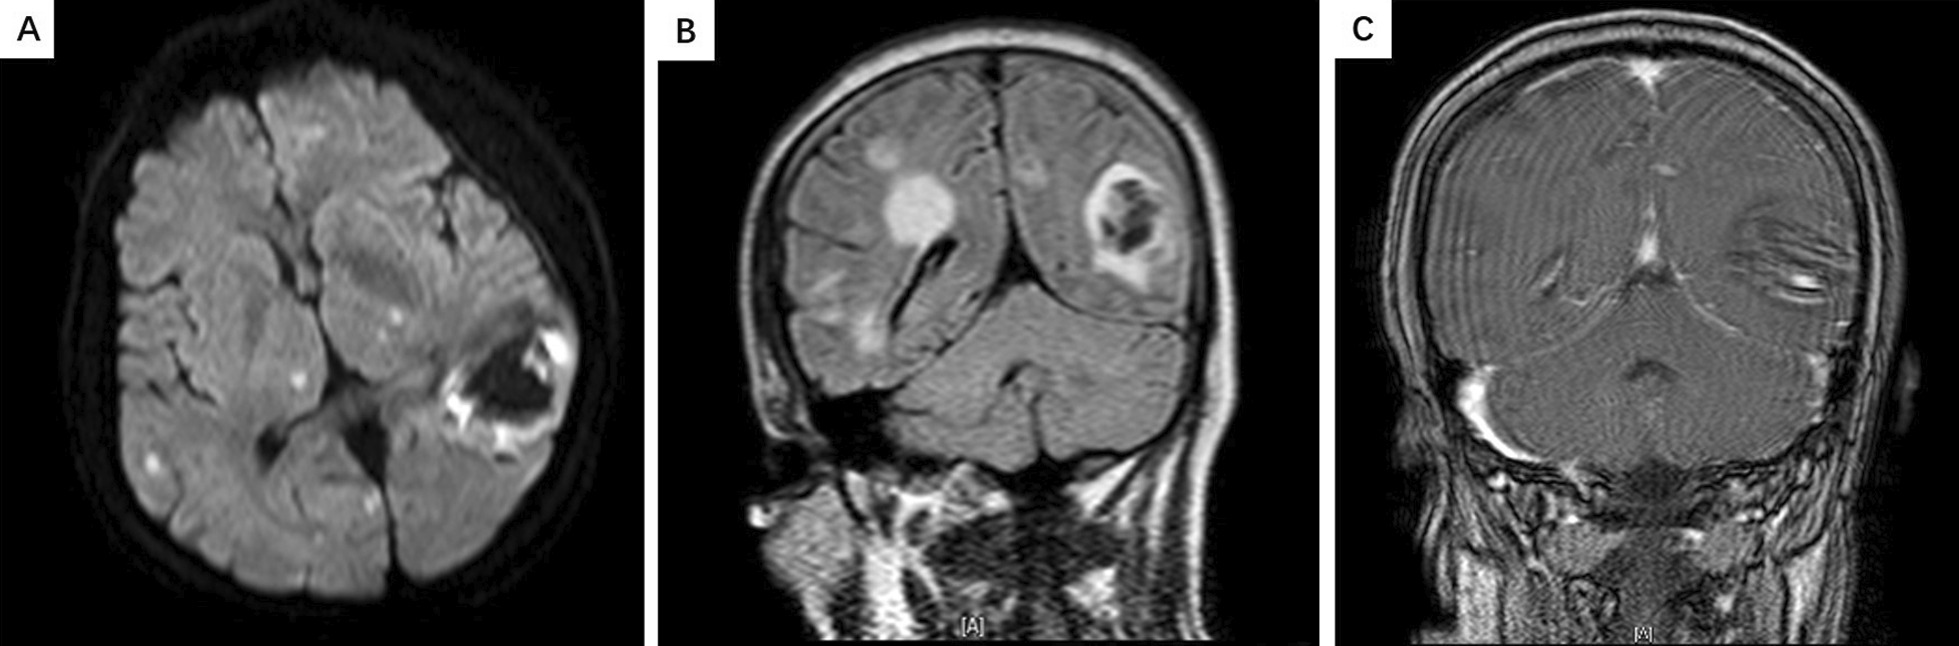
A Brain MRI showed patchy mixed signal shadows in the left occipital lobe, with a diameter of approximately 3.9 cm. B, C Short T1 signal shadows were visible, and patchy enhancement was observed. Multiple patchy slightly longer T2 signal shadows can be seen in both cerebral hemispheres. DWI phase shows high signal intensity, surrounding areas of oedema, and enhanced patchy enhancement
